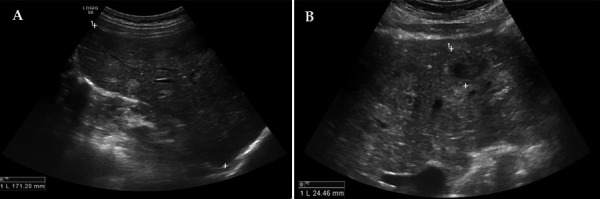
Abdominal ultrasound images.* Hepatomegaly and multiple variable echogenicity liver lesions (metastases) throughout all liver segments (the biggest ~25 mm in diameter) (A, B).
